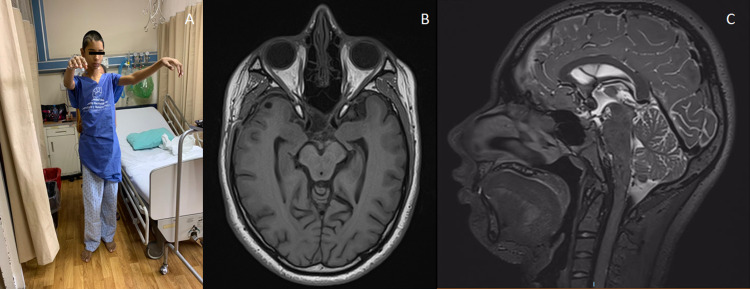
Fourth day post-op. A, Patient showing good surgical recovery. B-C, Axial MRI T1-weighted and Sagittal T2.weighted images showing complete cavernous malformation’s resection.
